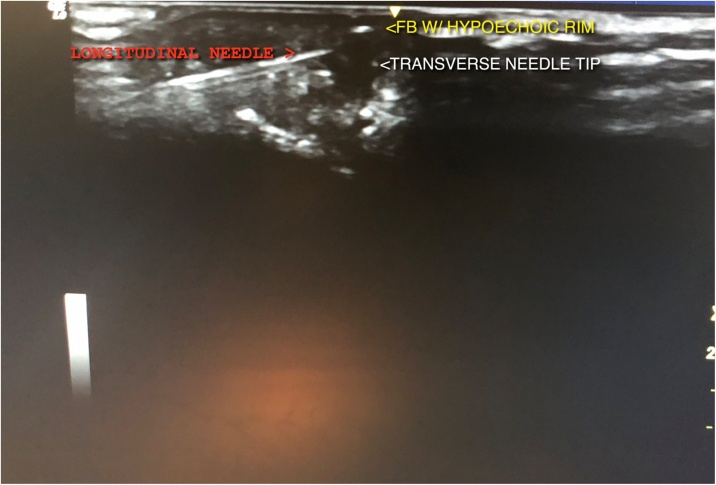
Identification of a hyperechoic foreign body on ultrasound with surrounding granulation tissue. Needle tips placed deep to foreign body, preventing displacement. Tips of the transverse and longitudinal needles can be appraciated.
